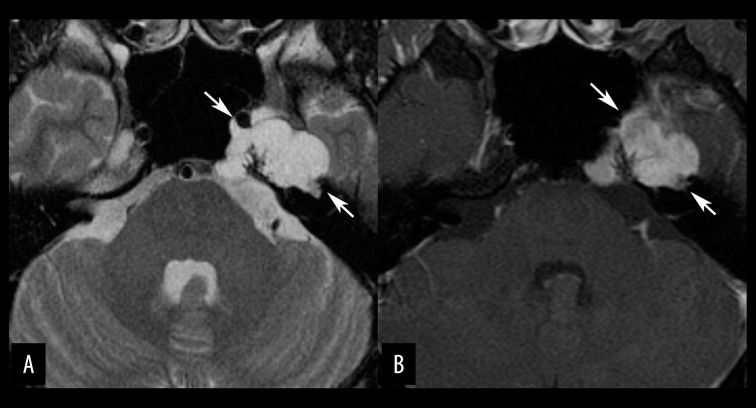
Chordoma in a 35-year-old man. (A) Axial, fat-suppressed, T2-weighted MR image shows a lobulated, very hyperintense mass (arrows) in the left petrous apex. The tumor extends to the left Meckel’s cave but the epicenter of the tumor lies in the left petrous bone. (B) Axial, contrast-enhanced MR image with fat suppression shows rather homogeneous, strong enhancement of the tumor (arrows). This is a case of lateral variants of chordoma. About one-third of skull base chordomas occur at off-midline positions.
